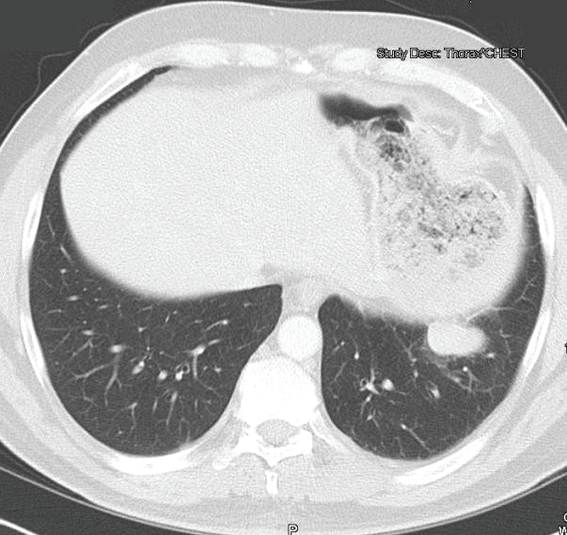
CT scan of the chest and abdomen revealed presence of a 3.4 cm mass adjacent to the left hemidiaphragm.
